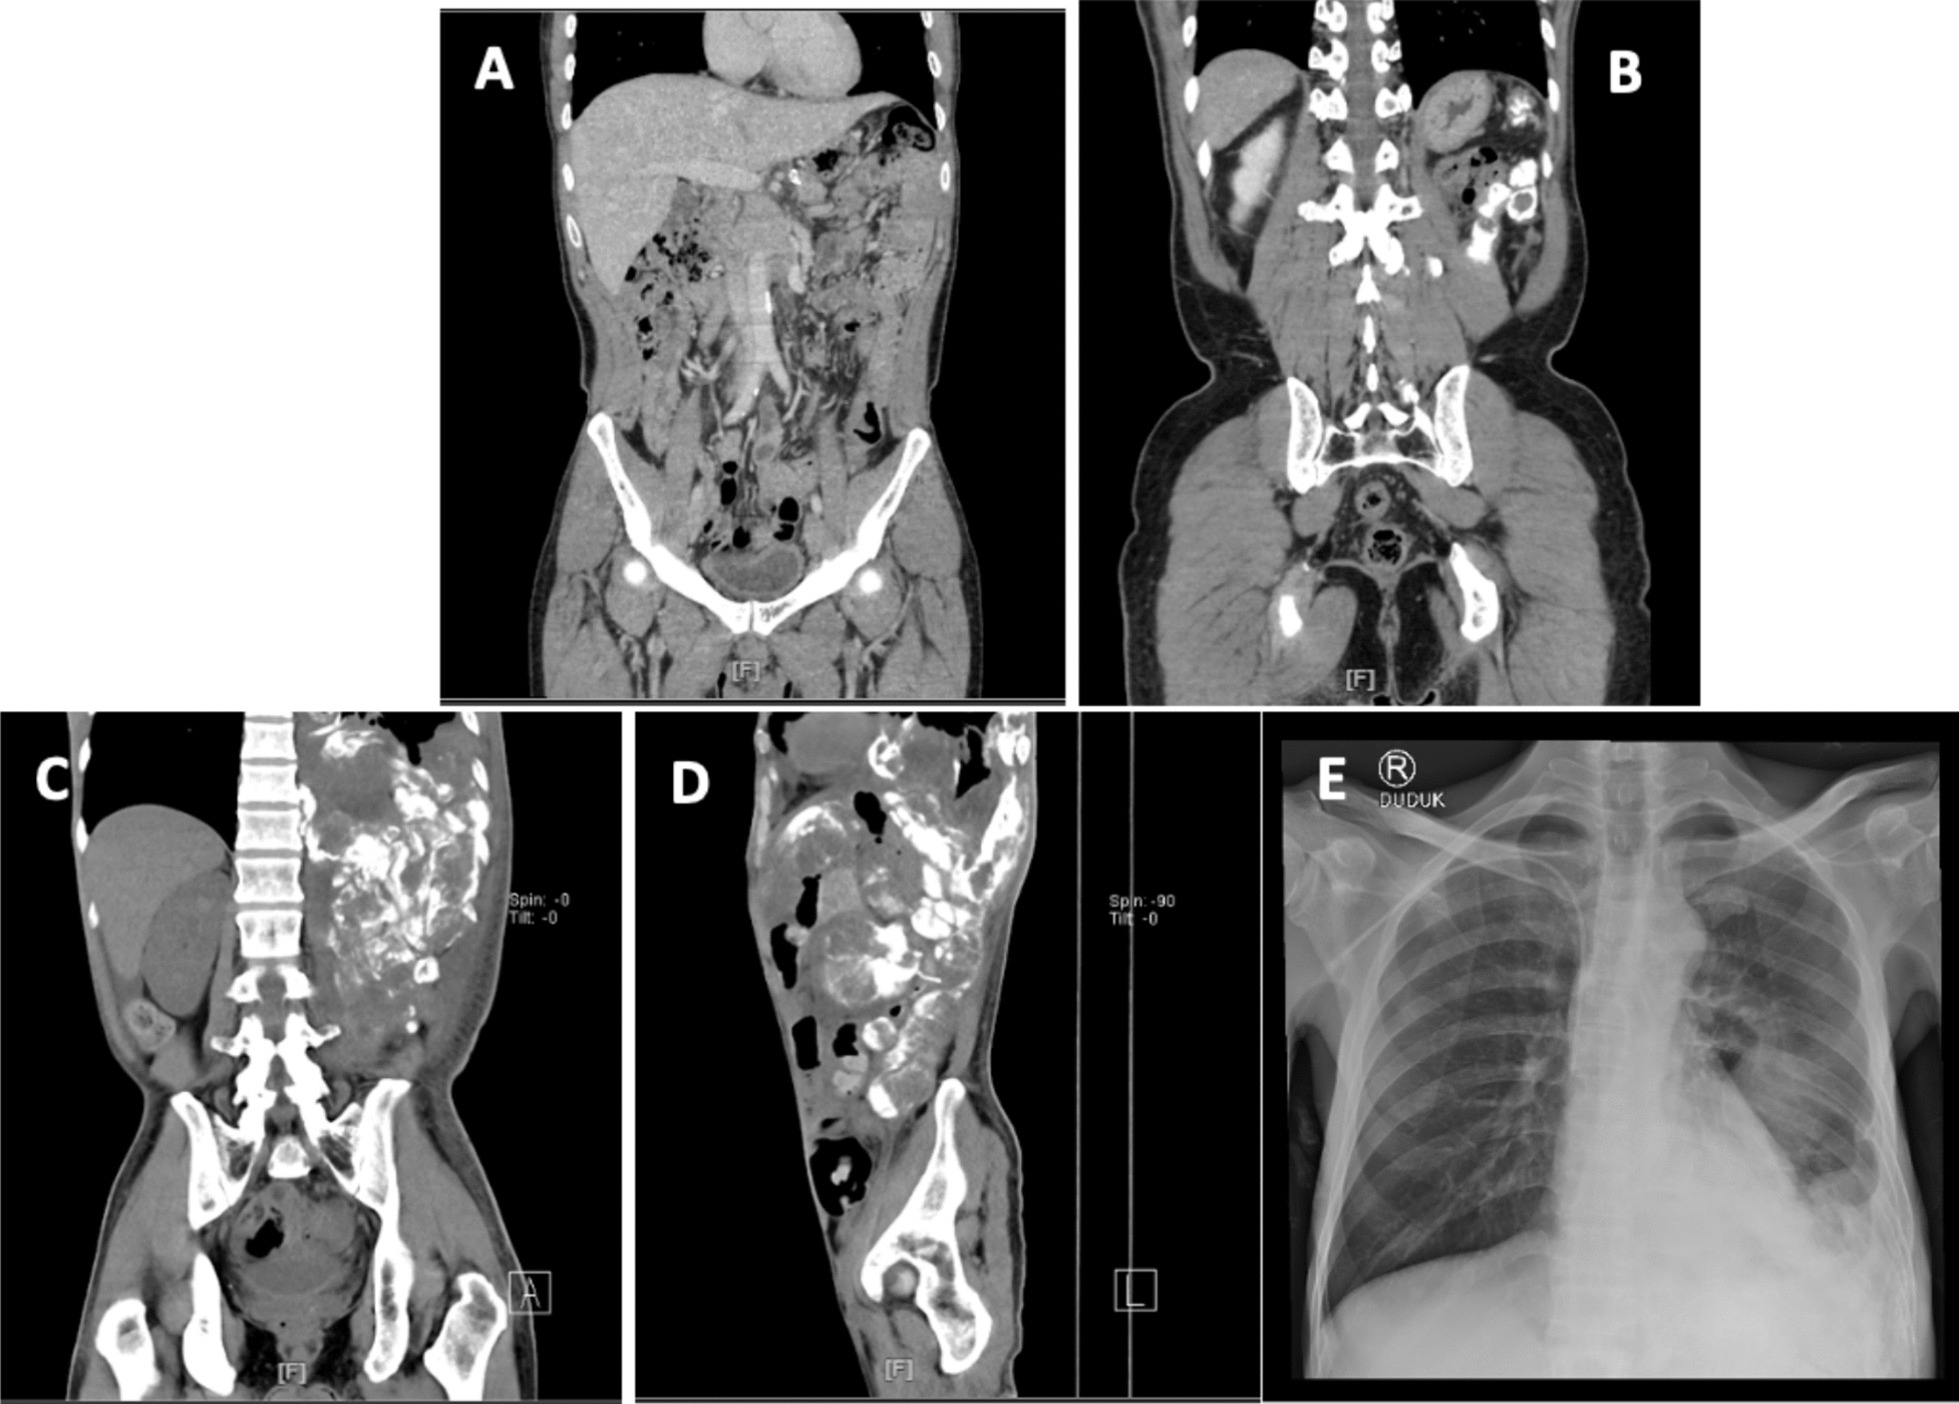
Routine scheduled imaging follow-up after surgery. A Computed tomography—abdomen and pelvis without contrast, coronal view (8 months). B Computed tomography—whole abdomen with contrast, coronal view (12 months). C Computed tomography—whole abdomen without contrast, coronal view (19 months). D Computed tomography—whole abdomen without contrast, sagittal view (19 months). E Chest X-ray, AP (19 months)
